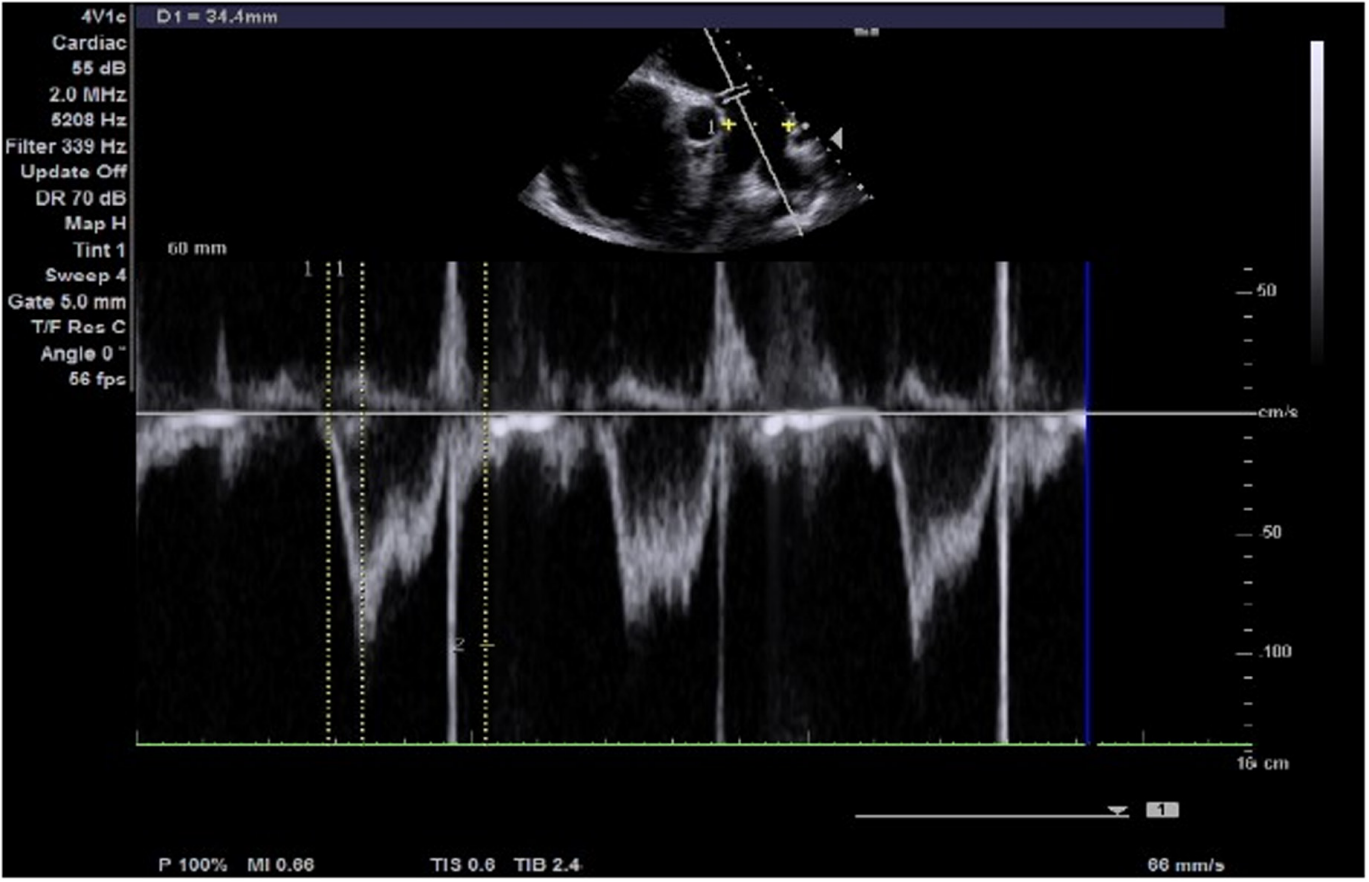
Pulse wave Doppler image showing dilated pulmonary artery of diameter (D1) 34.4 mm, normal pulmonary valve peak gradient of 1 m/s, and shortened pulmonary artery acceleration time of 96 ms.
